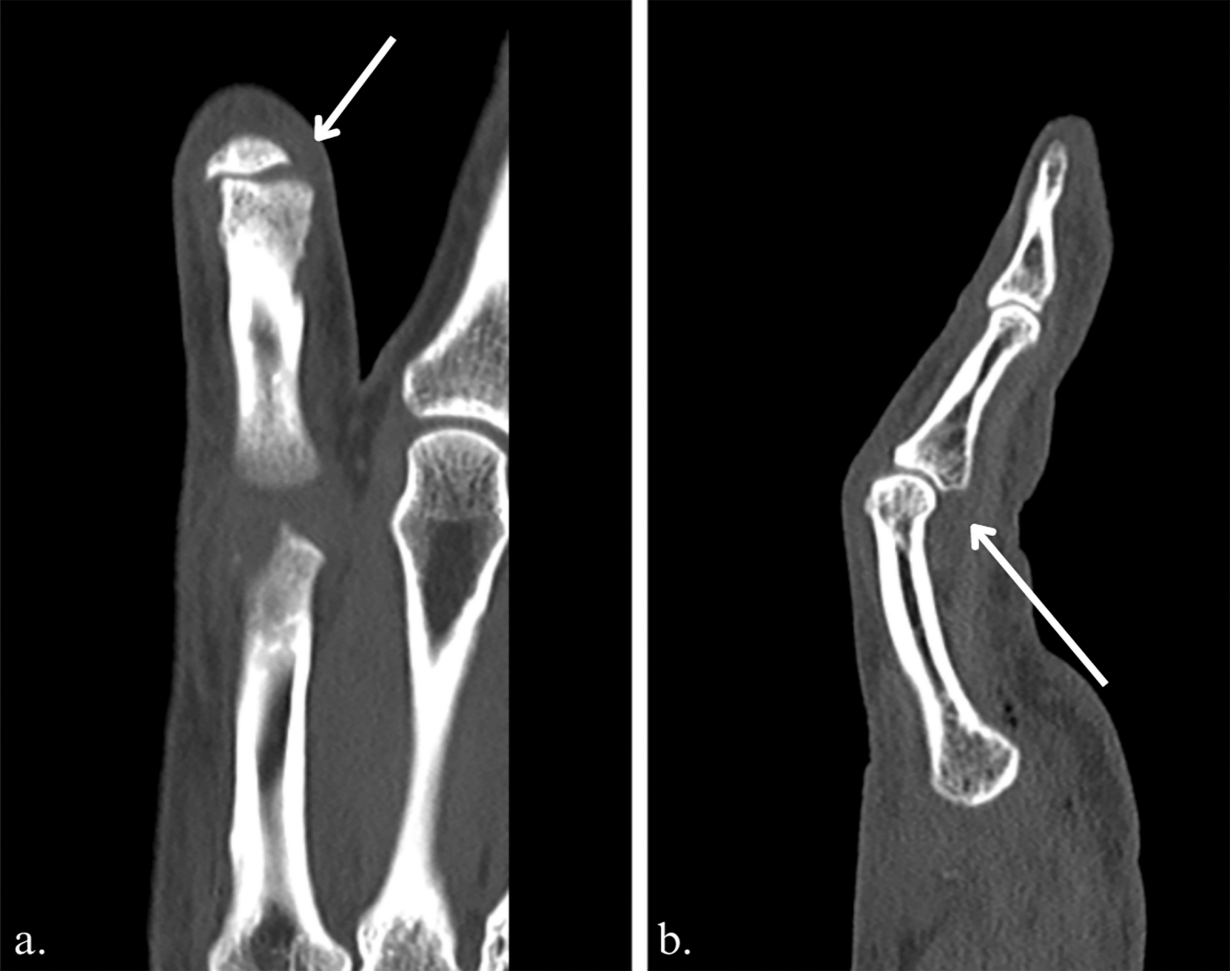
CT scan showing a (a) radial gap of the proximal interphalangeal (PIP) joint (white arrow) suggesting an interposition and on (b) the flexum (white arrow).
